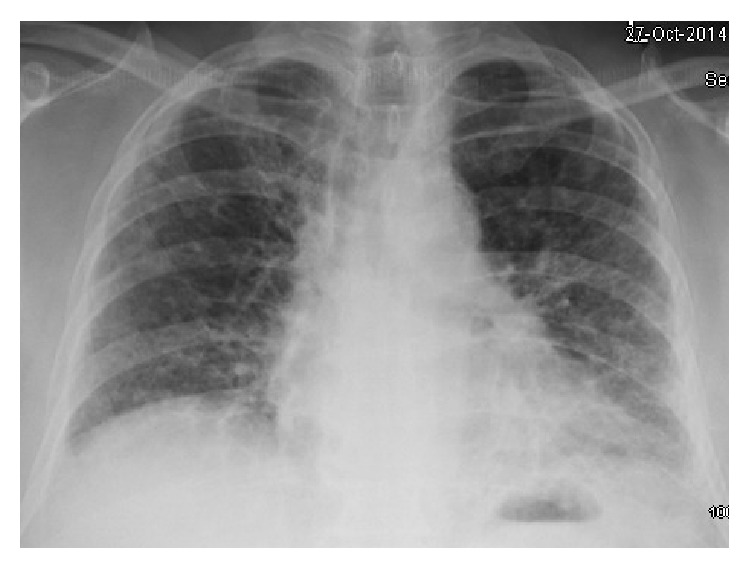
Chest X-ray taken 6 months prior to the day of admission showing mild hazy changes in bilateral lung fields.
